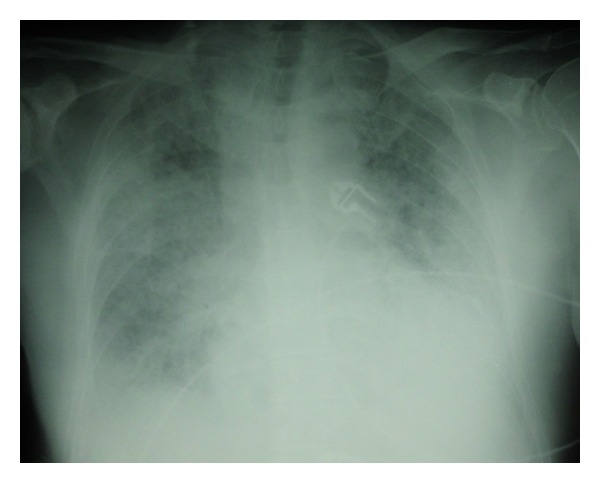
Postero anterior radiograph of the chest obtained on admission.
